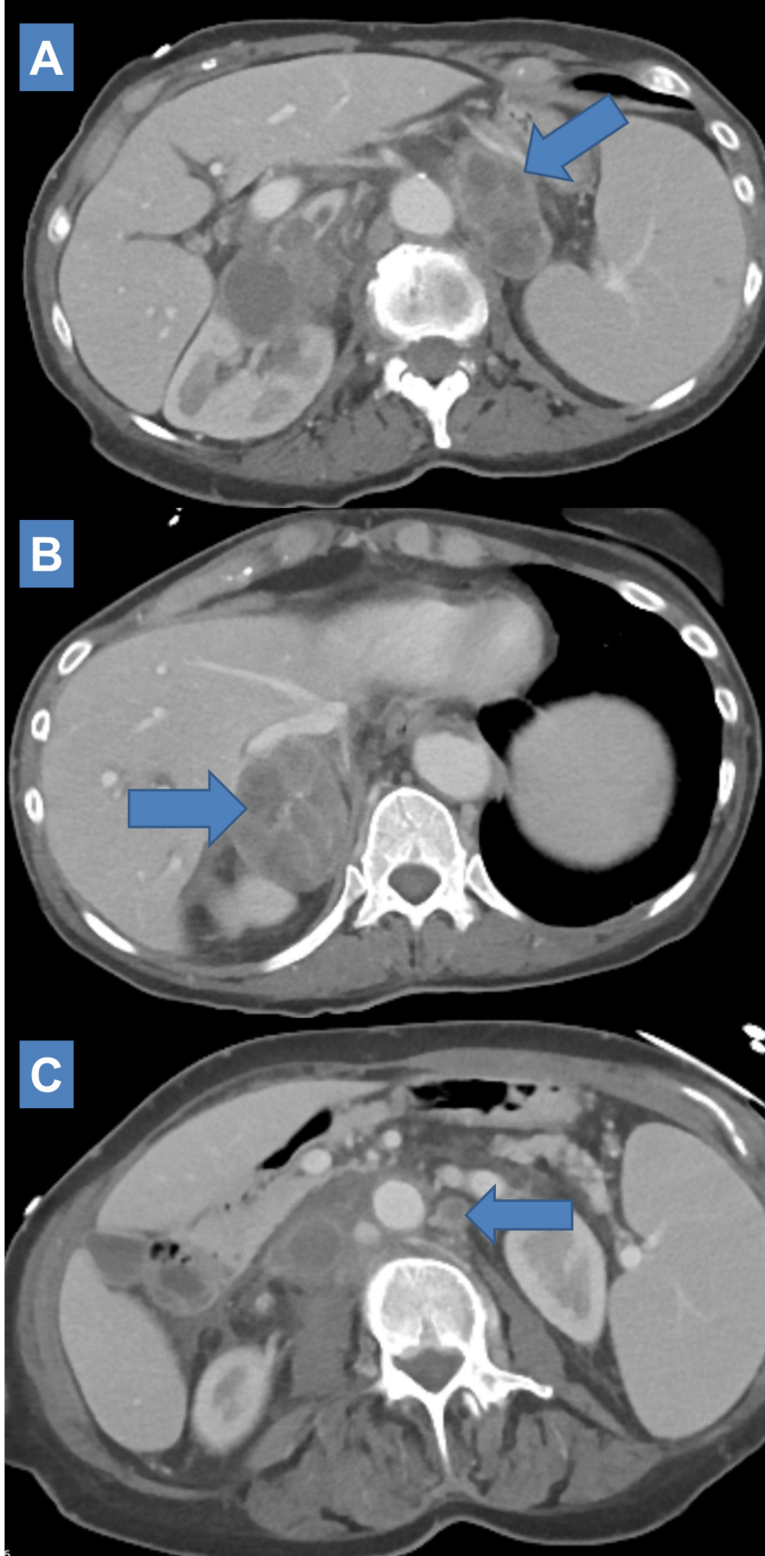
Six-month post salvage SBRT CT imaging scan showing progressive increase in size of left adrenal metastasis to 6.0 cm x 3.0 cm (A: Axial) and right adrenal metastasis to 6.4 cm x 3.5 cm (B: Axial) with the right adrenal metastasis evidencing local invasion (liver, kidney, IVC) and essentially stable size of left para-aortic lymph node (C: Axial). Nivolumab maintenance was held briefly during this time. SBRT: Stereotactic body radiation therapy; CT: Computed tomography; IVC: Inferior vena cava.
